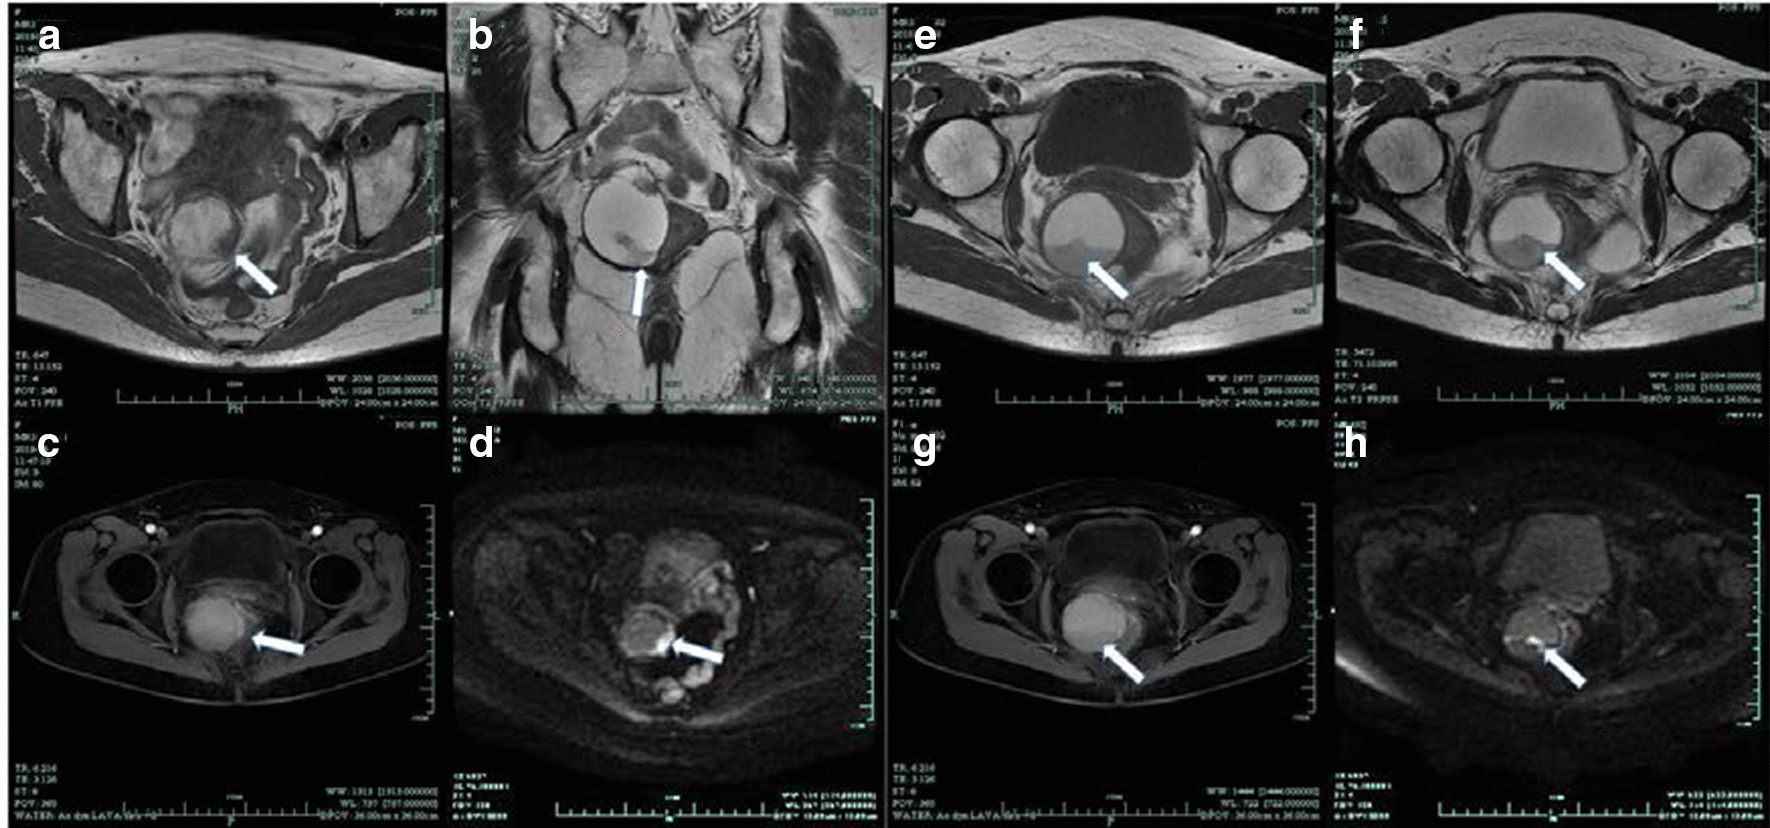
The magnetic resonance imaging findings of the mass. a–d The MRI imaging findings of the mural nodule of the tumour. e–h The MRI imaging findings of the septal nodule of the tumour. a and e T1-weighted MR images; b and f T2-weighted MR images; c and g on Gadolinium-enhanced T1-weighted MR images at arterial phases, the mural and septal nodule of the tumour showed heterogeneous enhancement. d and h The papillary excrescences of the tumour (arrow) showed high signal intensity on diffusion weighted imaging. The pathological finding was high-grade serous adenocarcinoma
